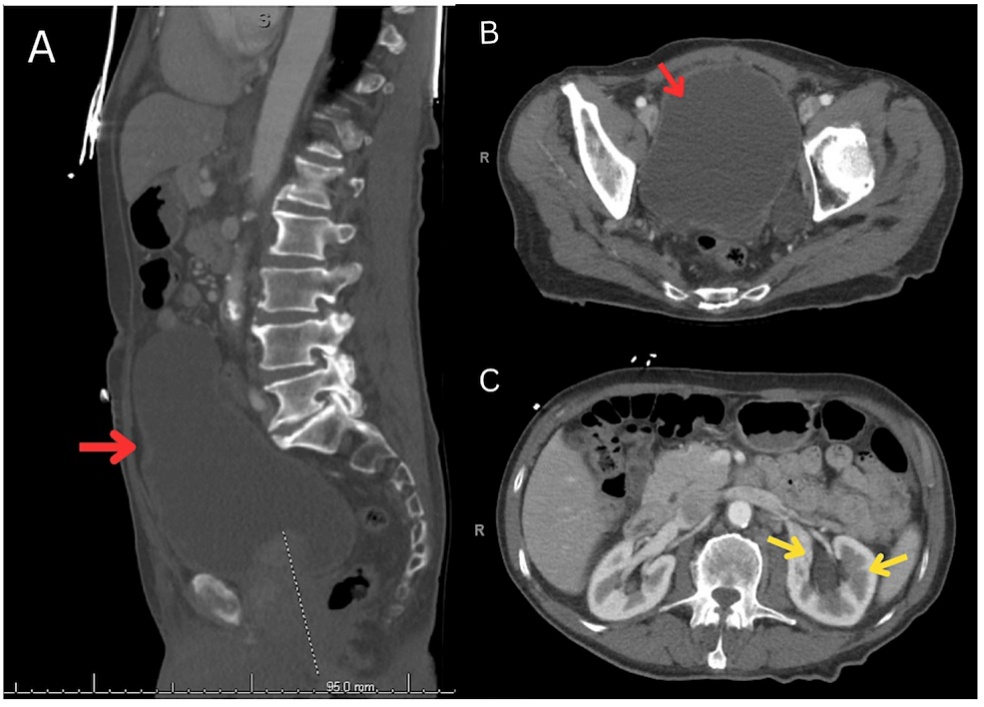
Abdominal CT scan. A. Sagittal view showing marked bladder distention (red arrow). B. Axial plane with markedly distended bladder (red arrow). C. Axial plane with moderate left hydroureteronephrosis (yellow arrows) and mild right hydronephrosis.
